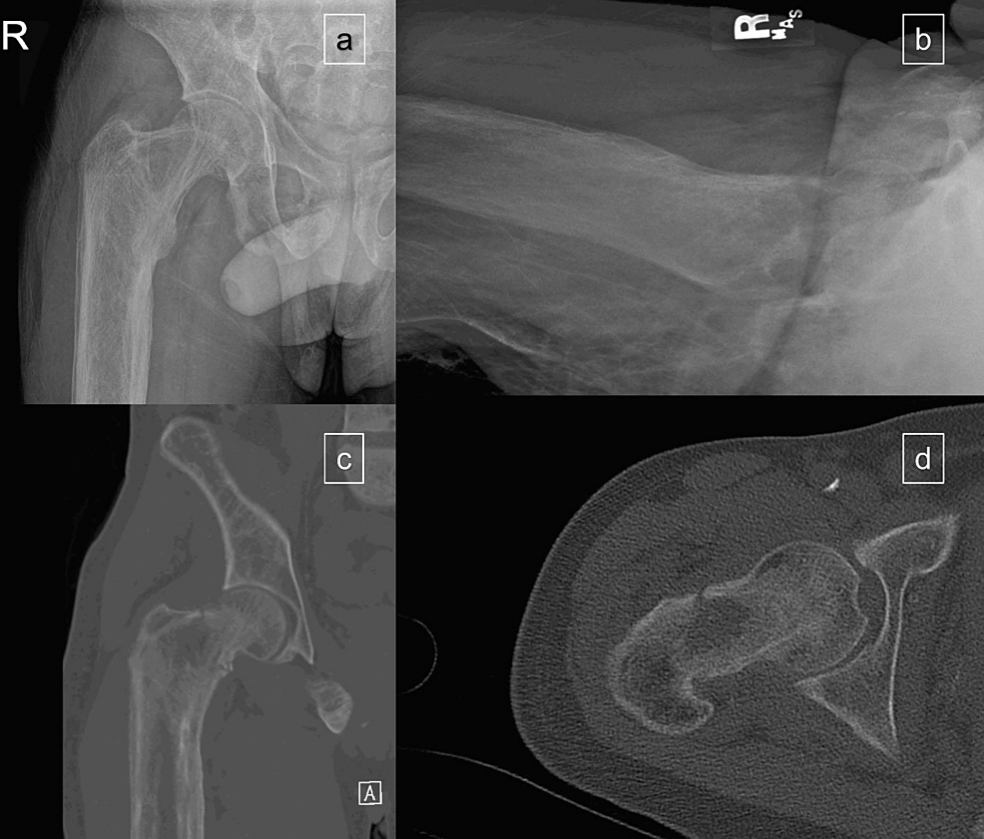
Preoperative radiographs (a and b) and CT scans (c and d) of a right basicervical (OTA/AO 31-B2.1) femoral neck fracture. Note the osseous abnormalities resultant from the patient’s renal osteodystrophy.
